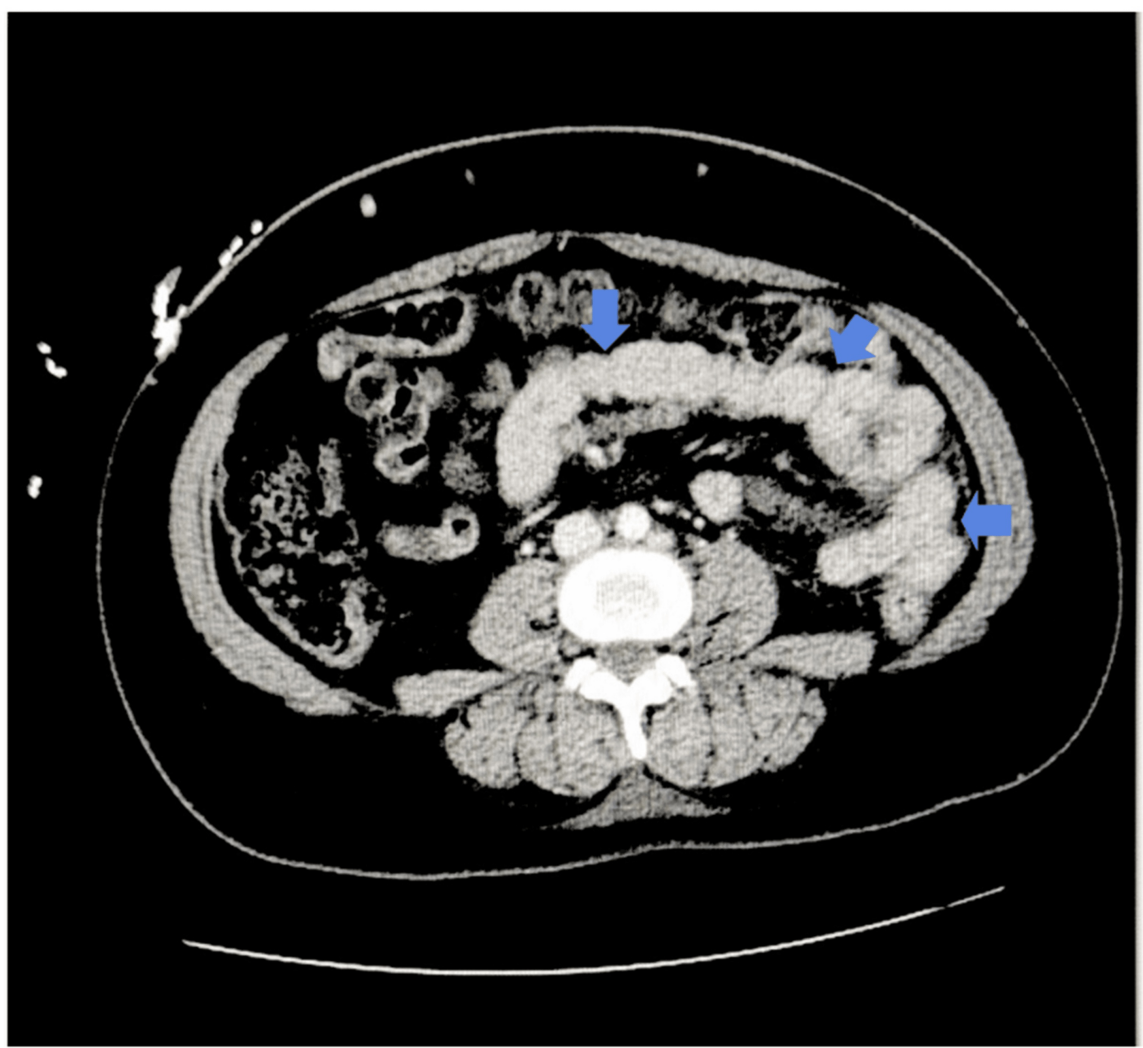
Axial CT image demonstrating peripancreatic fluid in acute pancreatitis. Axial contrast-enhanced CT image of the abdomen demonstrating inflammatory changes surrounding the pancreas. Peripancreatic fluid and inflammatory stranding are visible around the pancreatic body and tail (arrows), which are findings consistent with acute interstitial pancreatitis. CT: computed tomography
